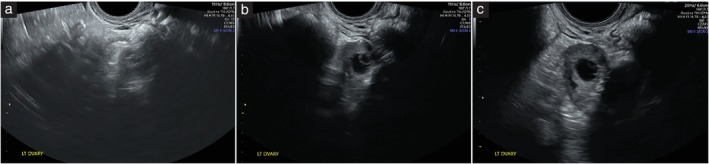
Example of ovarian ultrasound images with poor image quality (IQ) (score 2) (a), suboptimal IQ (score 3) (b) and optimal IQ (score 4) (c). A score of 1 was allocated to images deemed incorrect; these images were then rejected. (a) It is difficult to use this image for diagnosis as the ovary cannot be identified confidently, with the entire ovary not seen in the image, and optimization is poor. As the overall IQ is poor, a score of 2 is assigned. (b) The ovary can be seen. With the entire ovary visualized, optimization is satisfactory, but it could be improved, and diagnosis should be possible from the image. As the overall IQ is satisfactory, a score of 3 is assigned. (c) The entire ovary is documented clearly, optimization is good, and this image can be interpreted easily for diagnosis. As the overall IQ is good, a score of 4 is assigned. Lt, left.
